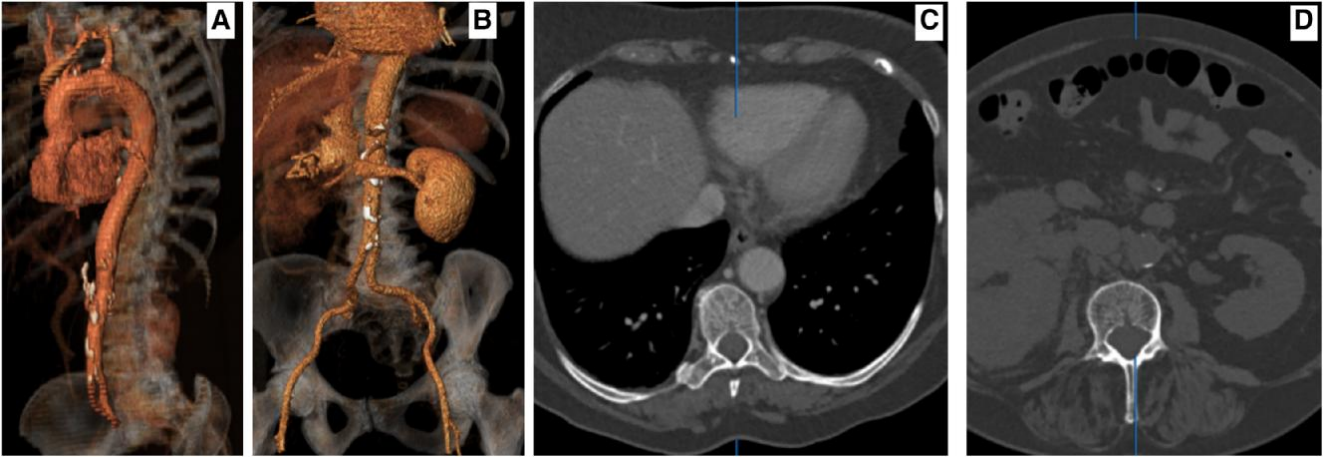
(*A*) Pre-operative to right nephrectomy, proximal three-dimensional imaging documenting a normal aorta. (*B*) Pre-operative to right nephrectomy, proximal three-dimensional imaging intravenous pyelogram revealing a tumour in the right kidney. (*C*) Pre-operative to right nephrectomy, axial computed tomography angiography documenting a normal aorta. (*D*) Pre-operative to right nephrectomy, axial intravenous pyelogram documenting a right kidney tumour.
